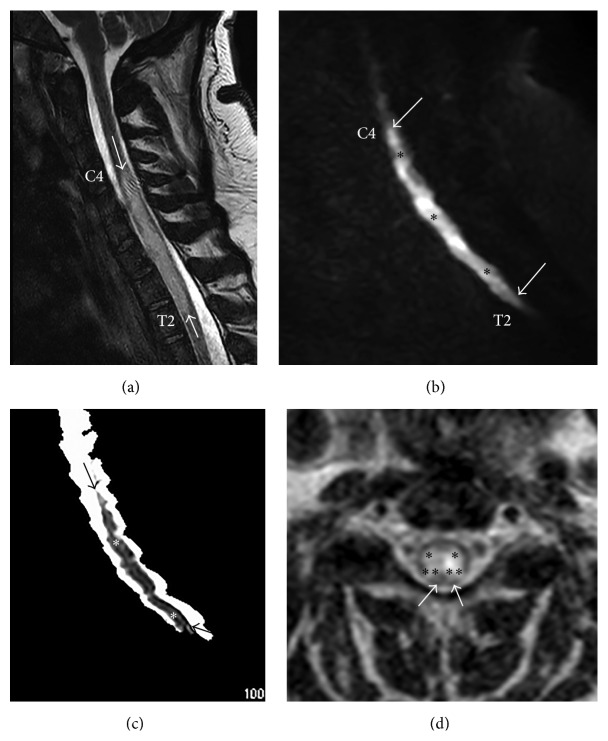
(a) Sagittal T2 weighted Fast Spin echo (FSE) MR image shows spinal cord expansion (swelling) with abnormal increased T2 signal extending from the C4 level to the T2 level (between the white arrows). (b) Sagittal DWI trace image shows abnormal increased signal intensity between C4 and T2, corresponding to the abnormal T2 signal noted on Sagittal T2 FSE imaging. (c) Sagittal apparent diffusion coefficient (ADC) map shows abnormal restriction of diffusion (dark signal in spinal cord indicated by arrows and asterisks) indicating that the increased signal on the DWI trace image represents diffusion restriction (not T2 shine through). (d) Axial T2 weighted FSE image at the C4 level shows cord expansion (swelling) and abnormal increased T2 signal within the swollen, edematous central gray matter (single asterisks indicate anterior horns and double asterisks indicate posterior horns of central gray matter). White arrows indicate uninvolved dorsal columns of the spinal cord.
